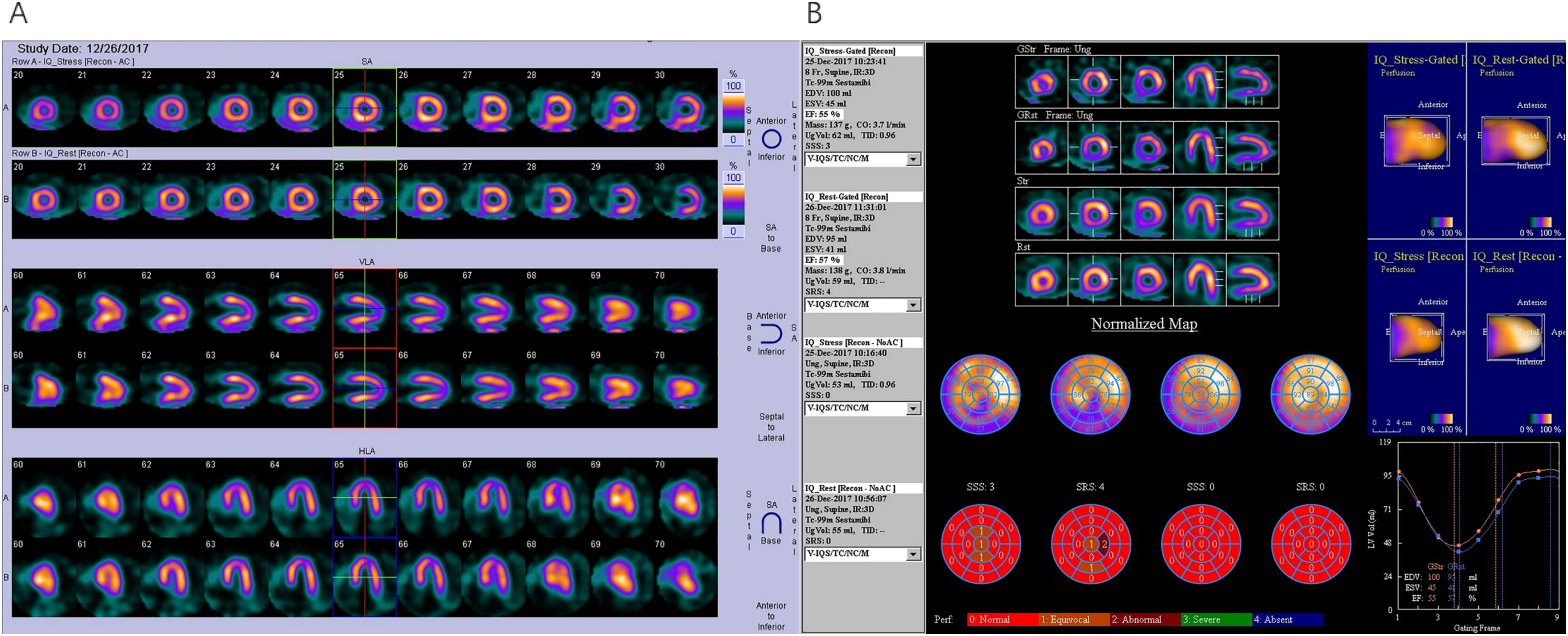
SPECT G-MPI in a 61-year-old male with CAD and with a history of PCI. (A) Perfusion imaging showing mild stress-induced ischemia in the apical inferior. (B) Analysis of cardiac function parameters revealed a LVEF_Stress_ of 55%, a LVEF_Rest_ of 57%, and a ΔLVEF = LVEF_Stress_ – LVEF_Rest_ = −2%. Subsequent coronary angiography revealed the absence of significant stenosis in the LM and LAD, patency of stent in LCX, and 100% occlusion of RCA. After a failed attempt of PCI in RCA, the patient was given medical therapy, and acute myocardial infarction was detected after 2.5 years of follow-up. PCI, percutaneous coronary intervention; LM, left main stem; LAD, left anterior descending artery; LCX, left circumflex artery; RCA, right coronary artery.
